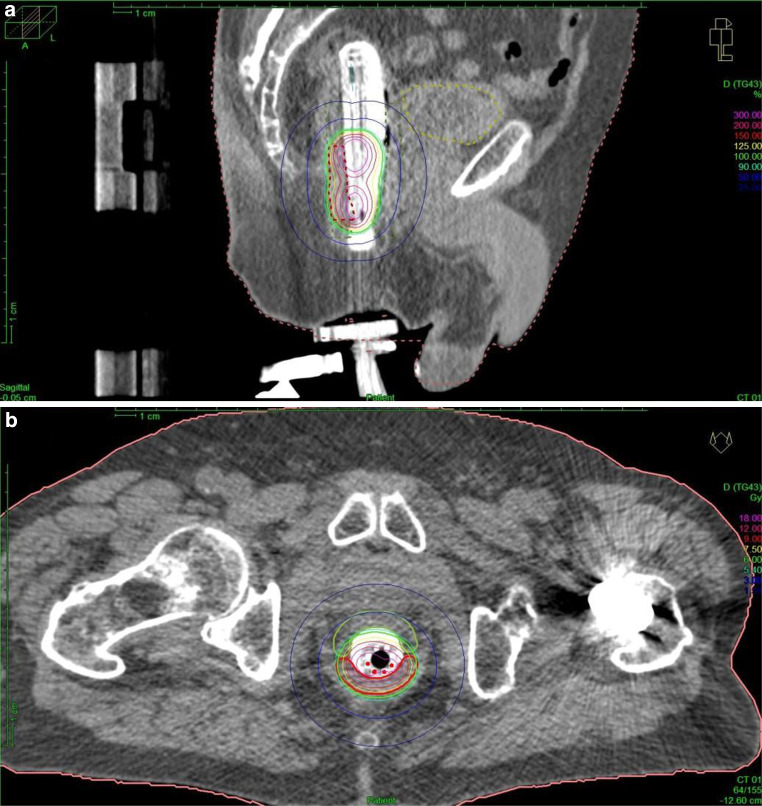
CT-based treatment planning of patient 6. Sagittal (a) and axial (b) images of CT-based treatment planning and distribution. A semicircular balloon filled with radiocontrast serves as a spacer to increase the distance between target volume and the contralateral rectal wall and ensures stable positioning and proper contact area. The applicator is additionally fixed to the treatment table by a specialized clamp. Isodose color code convention: *green* 6 Gy (100%), *red* 9 Gy (150%), *magenta* 18 Gy (300%, mucosa contact dose). Planning target volume (PTV) is also marked in *red*
